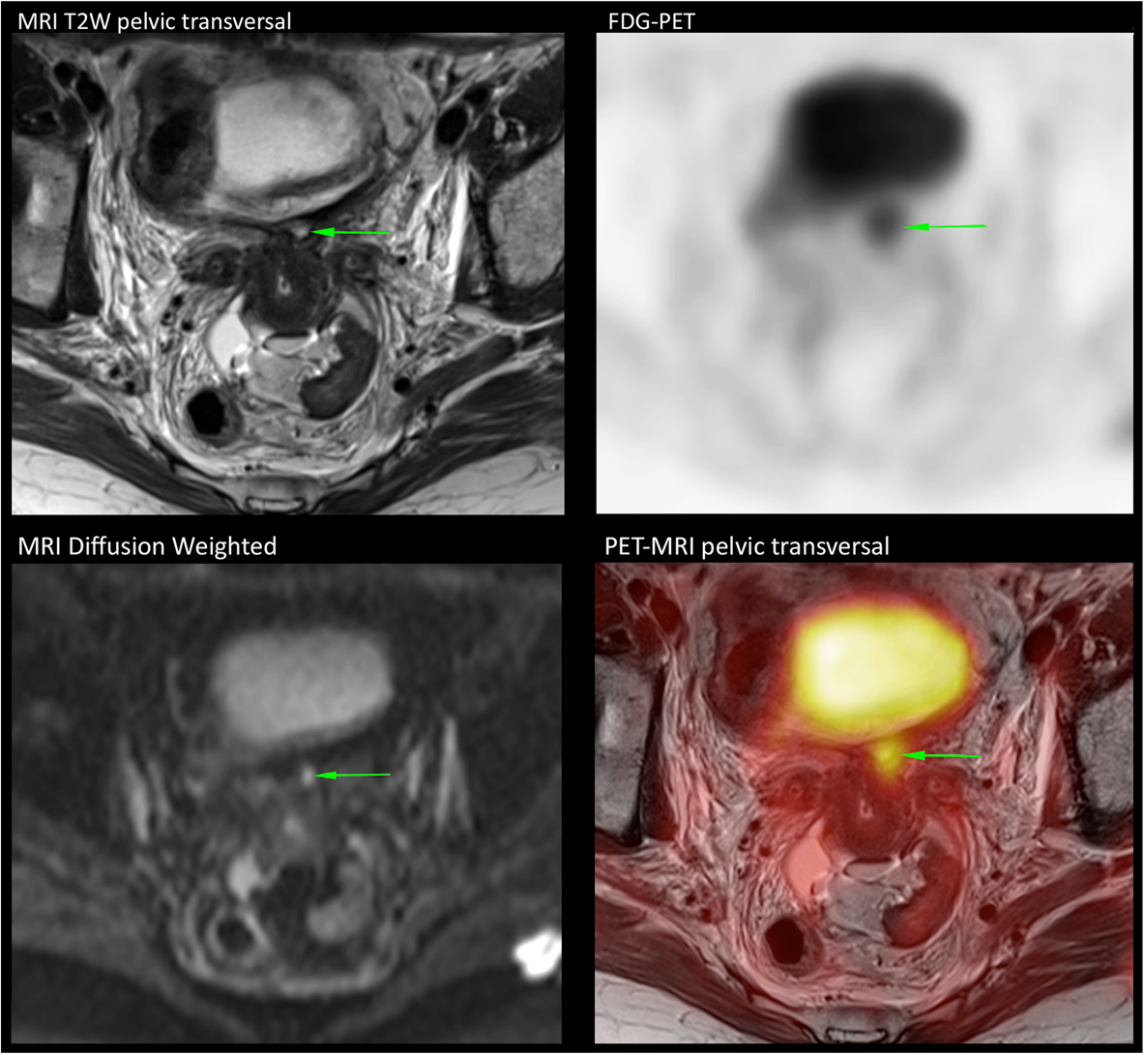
MRI T2W, diffusion-weighted MRI with ADC map, FDG-PET, and PET-MRI fusion showing local residual cancer (green arrow)
